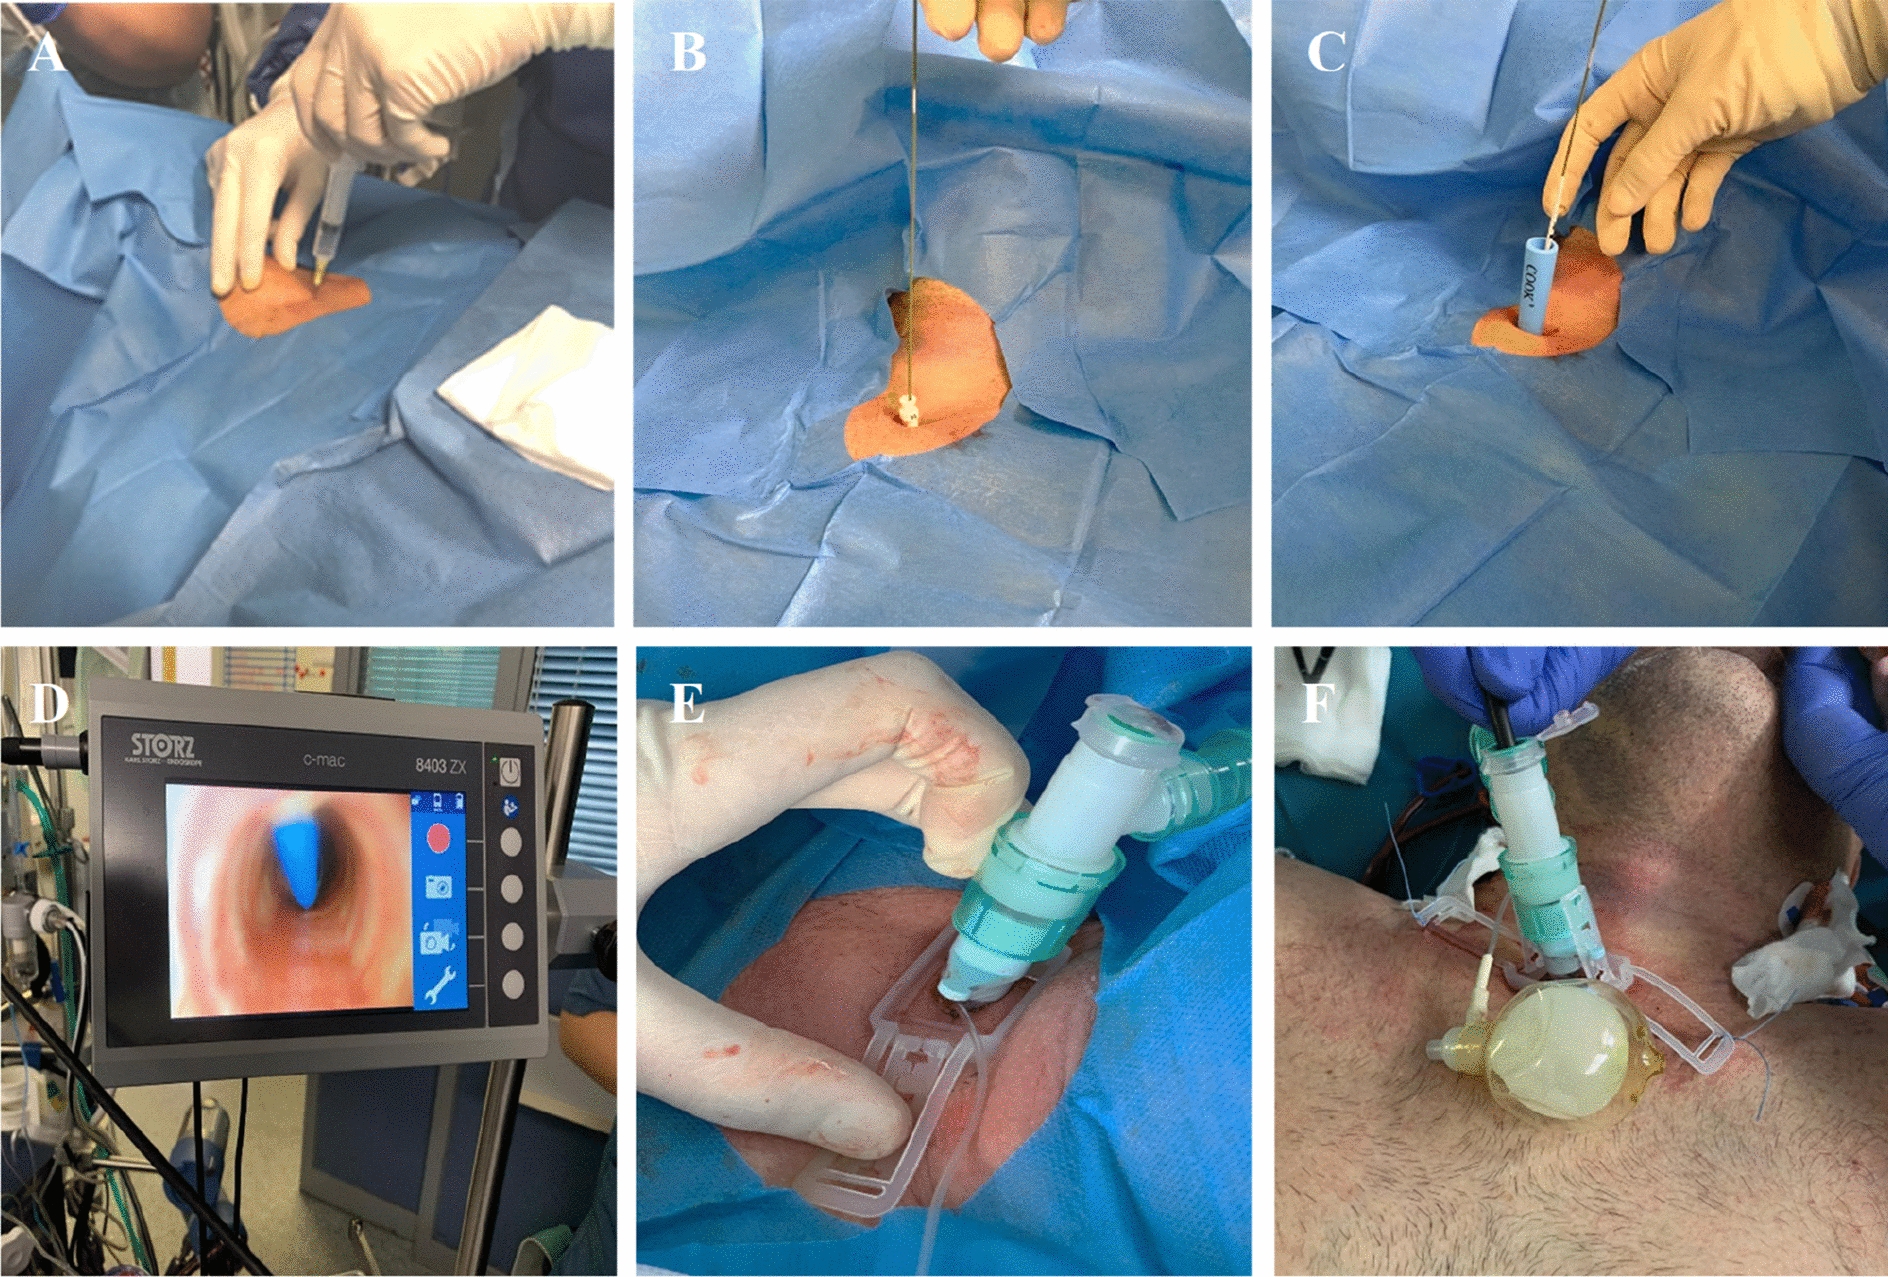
Percutaneous dilatational tracheotomy according to Ciaglia’s technique. Percutaneous dilatational tracheotomy according to Ciaglia’s technique with accompanying bronchoscopy: first, a colour Doppler ultrasound examination may be performed to ensure that no blood vessels run below the intended puncture site. After administration of local anaesthesia (A), median puncture of the trachea below the second or third tracheal clasp under bronchoscopic visualization and insertion of a Seldinger wire (B) is performed. The Seldinger guidewire is used for bougienage with different dilators (Ciaglia Blue Rhino® Set, Cook Medical) (C) under bronchoscopic visualization (D). Subsequently, the tracheal cannula is inserted along an introducer and—after bronchoscopy-guided confirmation of correct localization of the cannula within the trachea and subsequent cuff inflation—connected to the respiratory system (E). Final bronchoscopic position control of the tracheal cannula (F)
